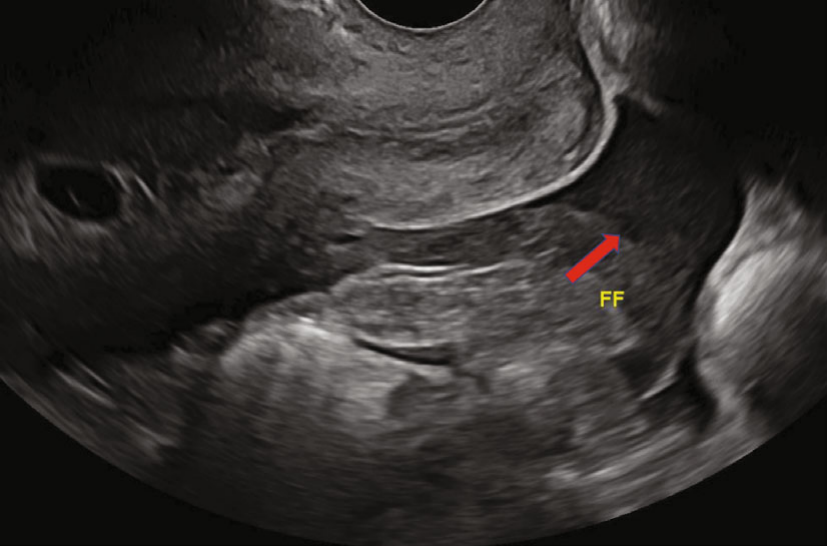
Sagittal transvaginal ultrasound image of the cervix with the posterior cul-de-sac with free fluid concerning for hemorrhage (red arrow).
